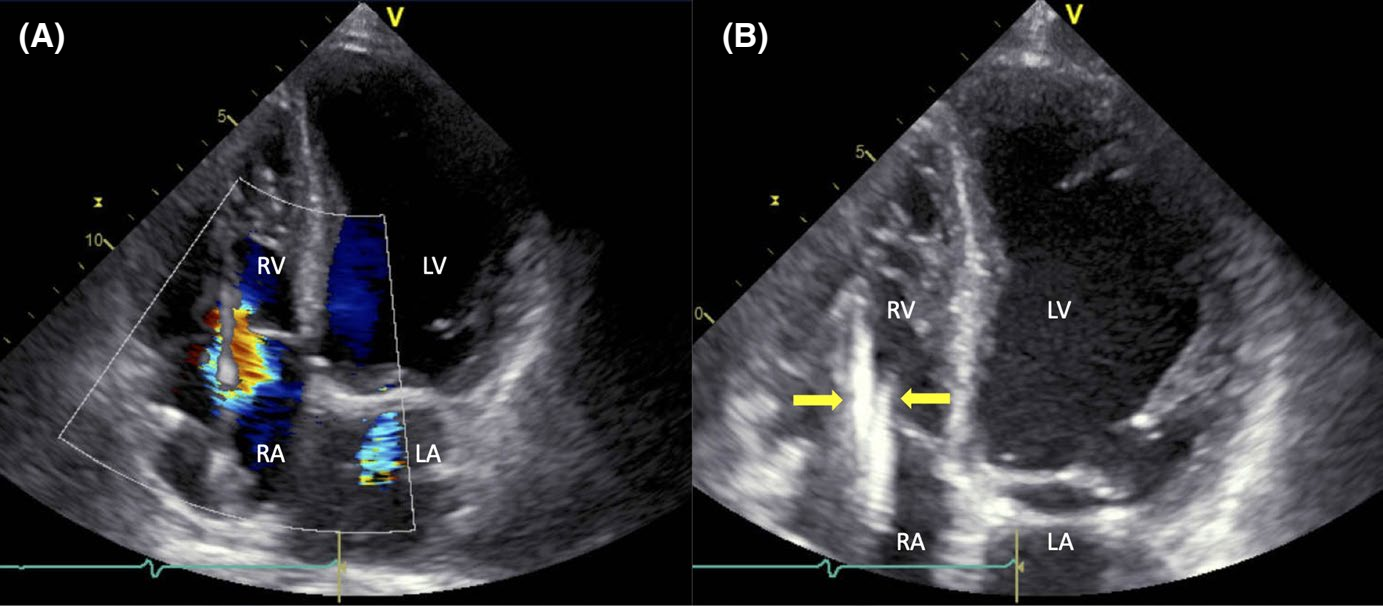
Transthoracic echocardiography showing severe tricuspid regurgitation (A) and two leads (arrows) entering the right ventricle through the tricuspid orifice (B). LA: left atrium, LV: left ventricle, RA: right atrium, RV: right ventricle
